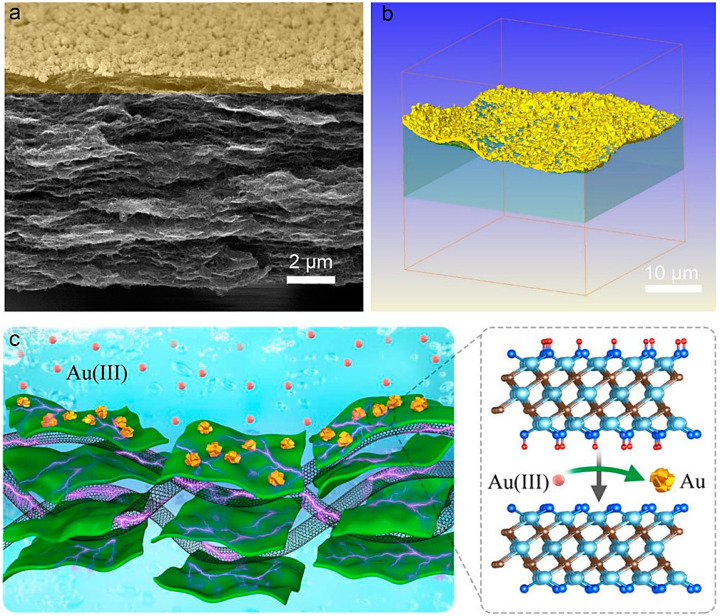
(a) SEM image of MXene/CNT-Au membrane (cross-sectional image). (b) Three-dimensional (3D) X-ray tomography (XRT) image of the membrane. (c) The proposed redox reaction mechanism of Au (III) rejection by the MXene-CNT hybrid membrane. Adapted from Ref [93] with permission. Copyright 2020 American Chemical Society.
